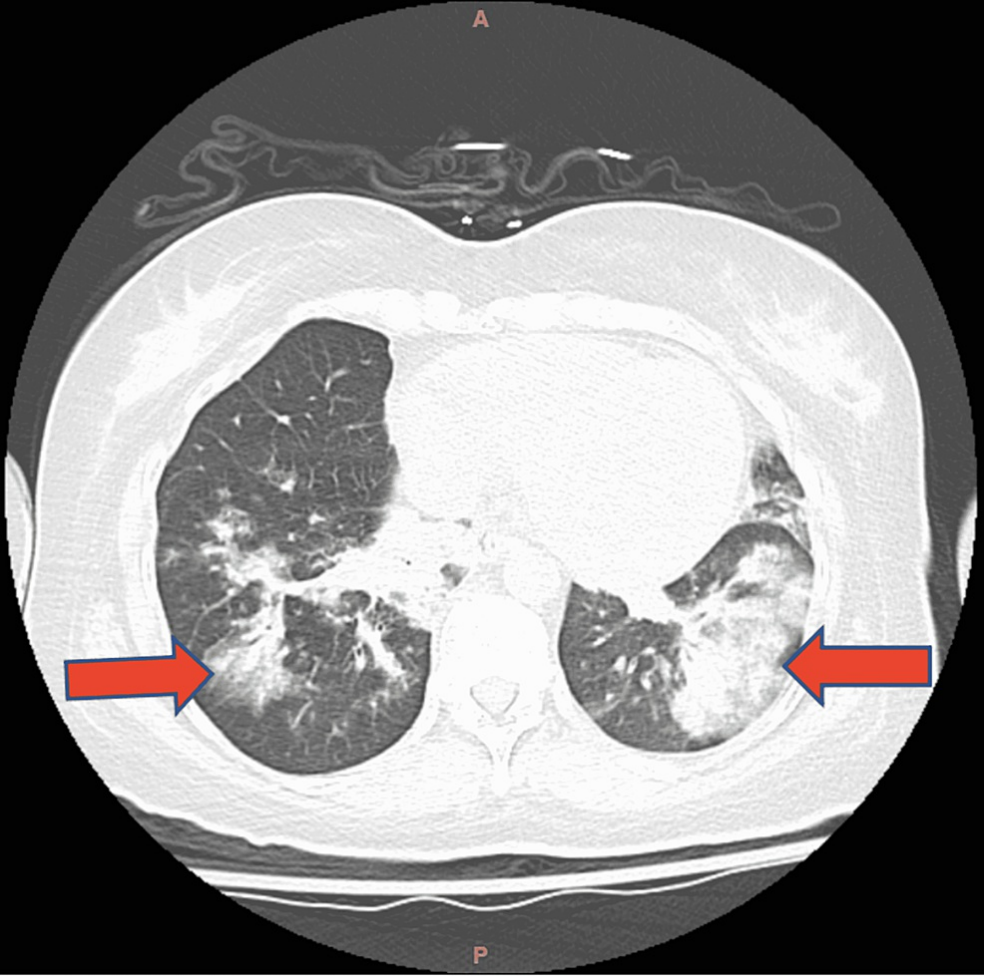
CT scan of patient's chest. Red arrows pointing at areas of disease consistent with bilateral pneumonia
